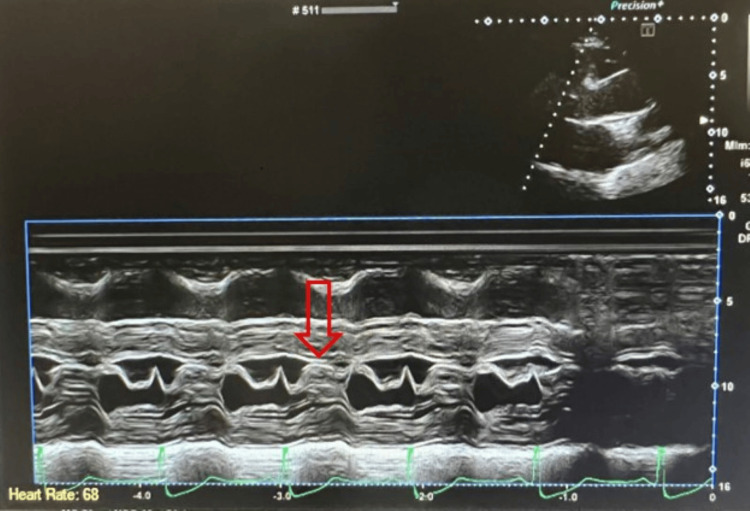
Transthoracic echocardiogram in M-mode showed systolic anterior motion of the anterior leaflet of the mitral valve (red arrow).
